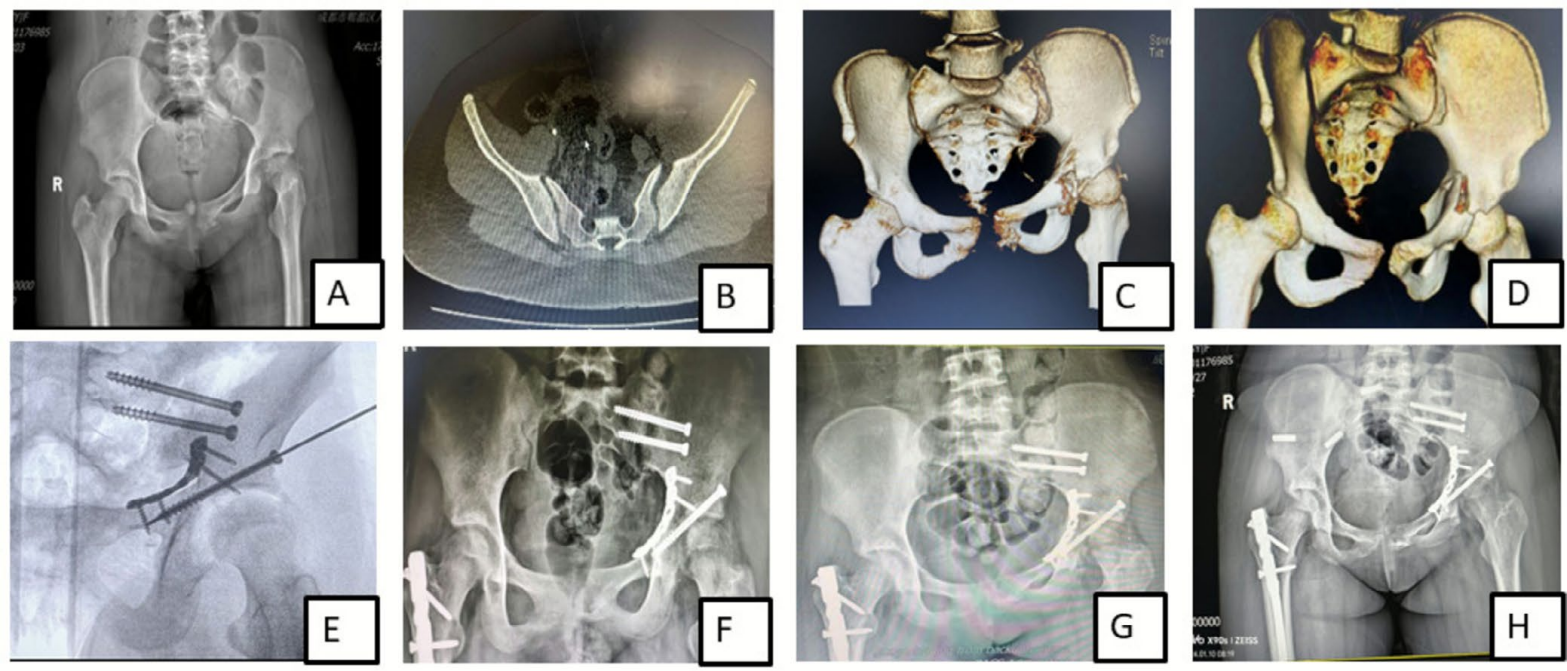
A 14‐year‐old female patient sustained an acetabular fracture after falling from a height. The post‐traumatic radiograph (AP view) showed a left acetabular fracture, left sacroiliac joint diastasis and right femoral shaft fracture (A). Preoperative CT scans revealed left sacroiliac joint disruption (B) and a minor pubic symphysis injury (C). 3D CT images from the left iliac view demonstrated a transverse fracture of the left acetabulum (D). Intraoperative fluoroscopic image displayed the fixation approach (E). Postoperative follow‐up radiographs were taken at 1 month (F), 6 months (G), and 1 year (H), respectively, showing good healing progression.
